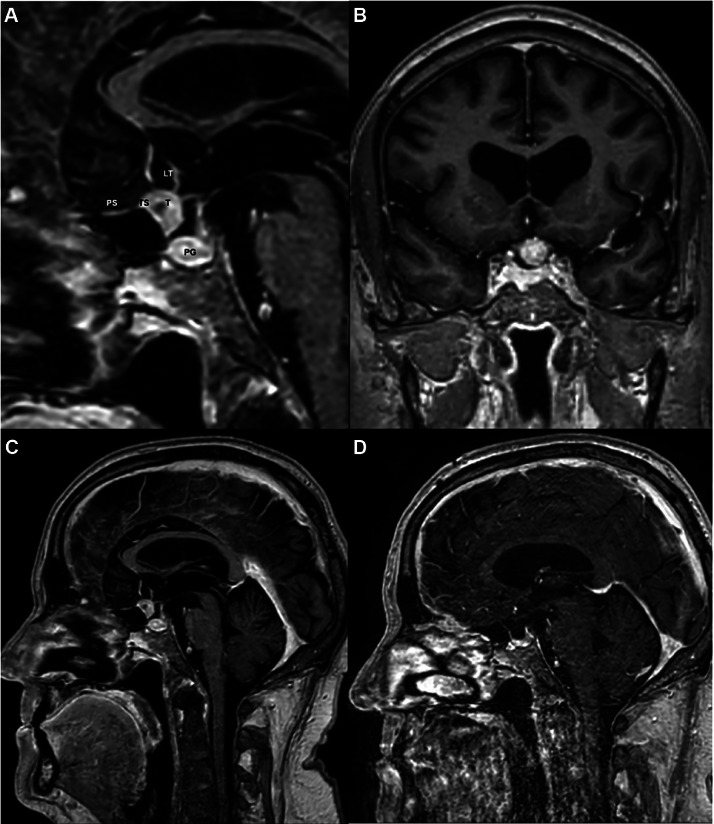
Preoperative and postoperative MRI scans of a symptomatic tuberculum sellae meningioma. (A) Sagittal magnified and (B) coronal contrast-enhanced T1-weighted MRI images demonstrating a well-circumscribed, contrast-enhancing lesion measuring approximately 1.2 cm at the tuberculum sellae bilaterally adjacent to the optic nerves, optic recess, lamina terminalis and anterior pituitary gland. (C) Preoperative and (D) postoperative sagittal contrast-enhanced T1-weighted MRI showing complete resection of the tuberculum sellae meningioma. LT, Lamina terminalis; PG, Pituitary gland; PS, Planum sphenoidale; T, Tumor; TS, Tuberculum sellae.
